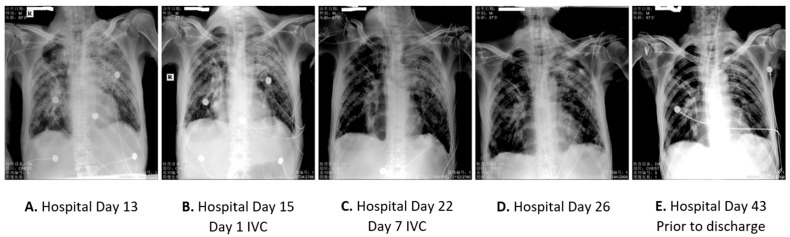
Chest X-ray images of patient 1. Hospital days 15–22 patient received IVC infusions. (A) Hospital Day 13 with worsening status and intubation. (B) Hospital Day 15 = Day 1 on IVC. (C) Hospital Day 22 = Day 7 on IVC. (D) Hospital Day 26 discharge from COVID-19 Unit. (E) Follow-up X-ray prior to hospital discharge.
